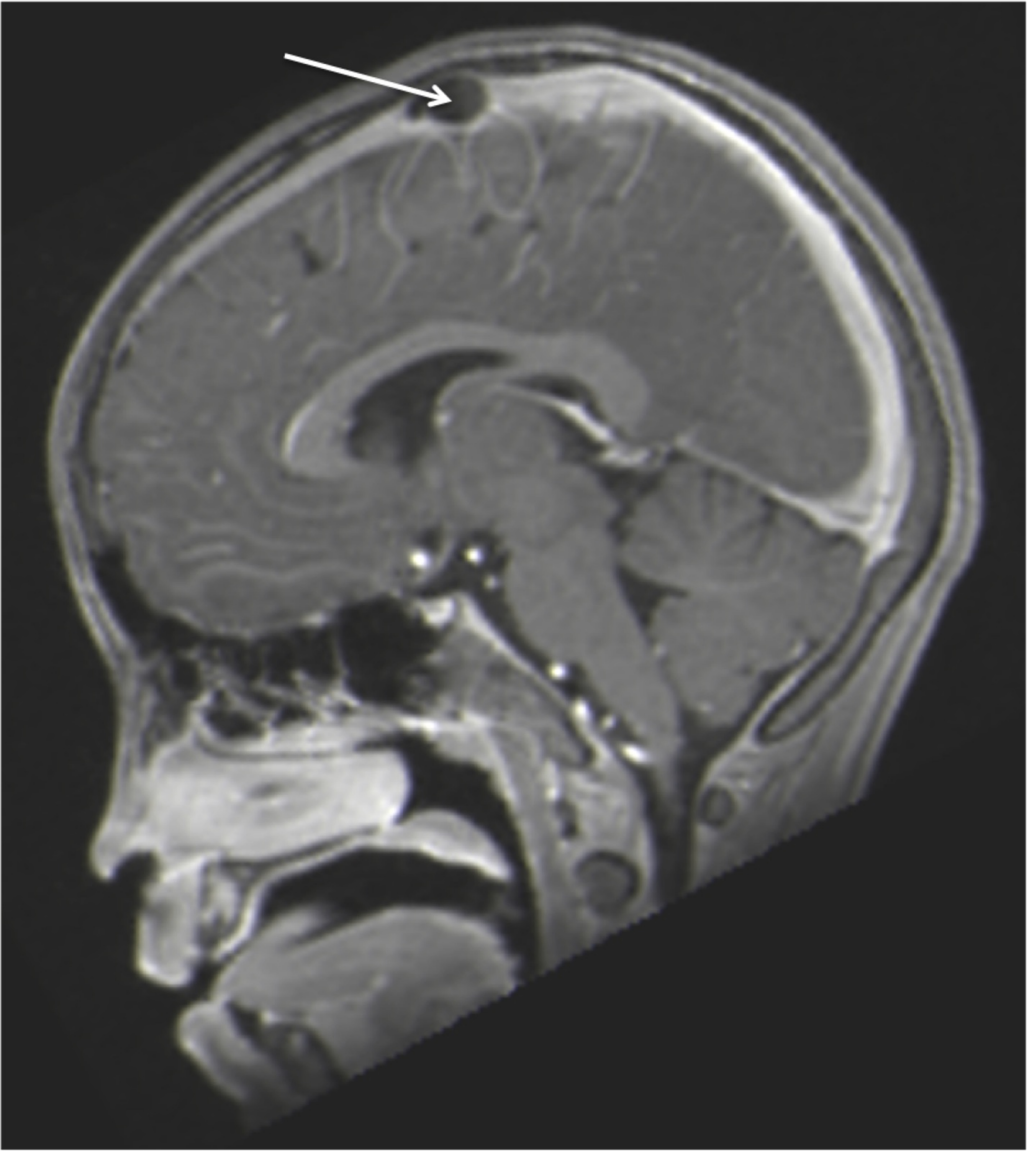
Sagittal contrasted MRI noting large mass (arrow) in the superior sagittal sinus.
